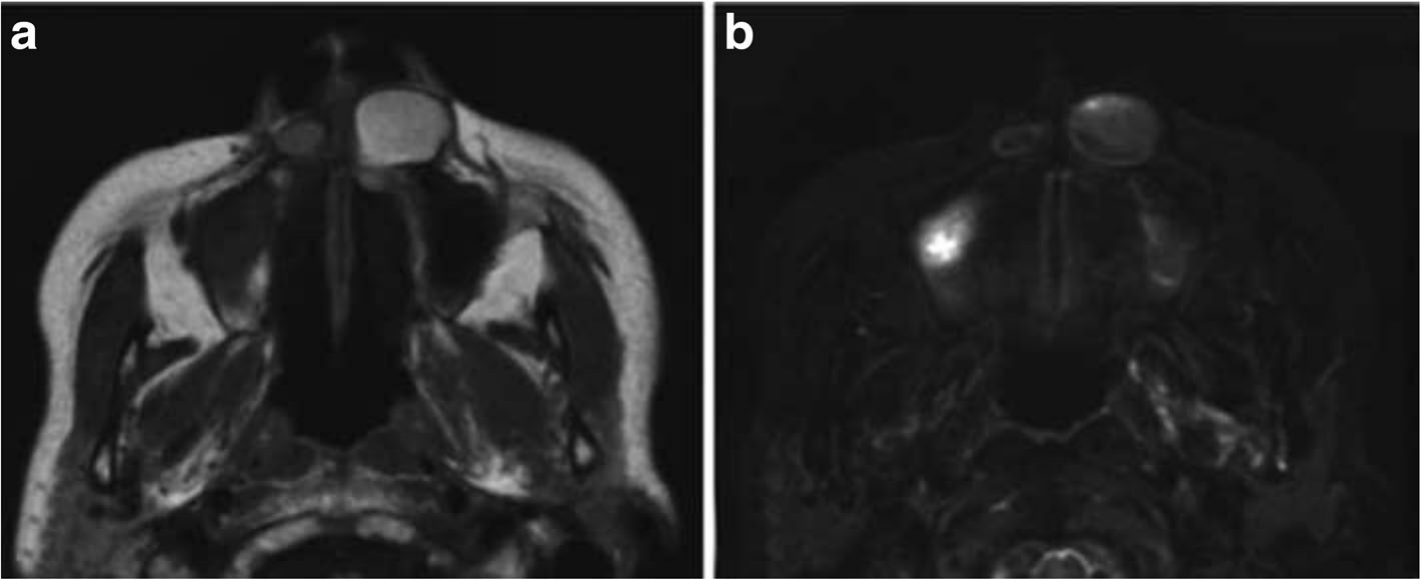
Magnetic resonance images obtained just before surgery. a. T1-weighted image. Oval lesions with high internal signal intensity inferior to the bilateral alar base. b. T2-weighted short inversion time inversion recovery image showing low signal intensity
